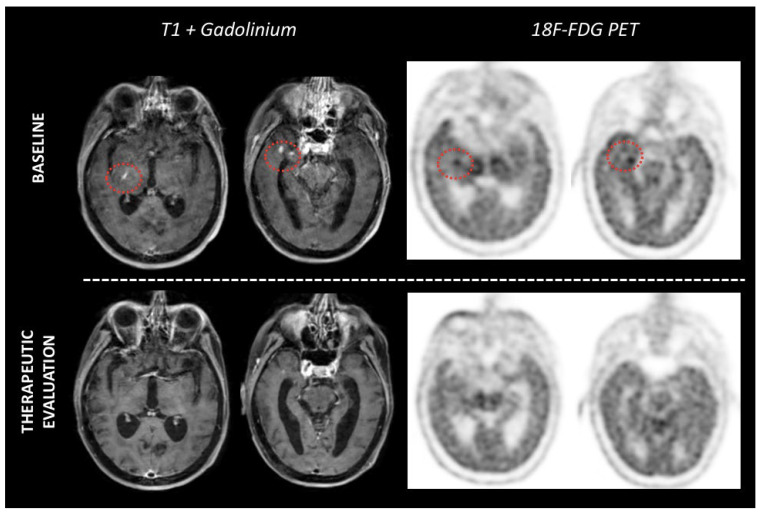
Example case of PET-MRI images of a lymphotamosis cerebri before and after Methotrexate therapy. Baseline images (top line) showed small nodular enhanced lesions anterior to the temporal horn of the right ventricle and a linear contrast-enhanced lesion at the posterior arm of the right internal capsule. ^18^F-FDG PET images revealed strong uptake consistent with these lesions. After three cycles of methotrexate treatment (bottom line), MRI images showed a partial response, while ^18^F-FDG PET images had already normalized.
